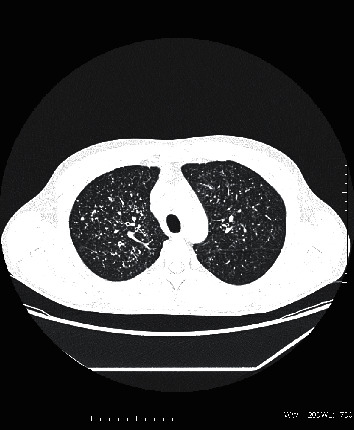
Axial chest computed tomography with the presence of innumerable small (2–4 mm) pulmonary nodules with a centrilobular predilection, consistent with miliary tuberculosis.
